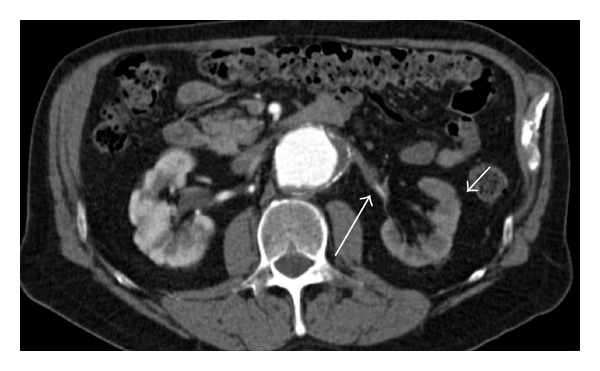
Preoperative CTA showing left kidney with preexistent occluded renal artery and contrast-enhancement in the cortical area (cortical rim sign, small arrow) with distal renal artery perfusion suggesting collateral (perirenal) flow (large arrow).
